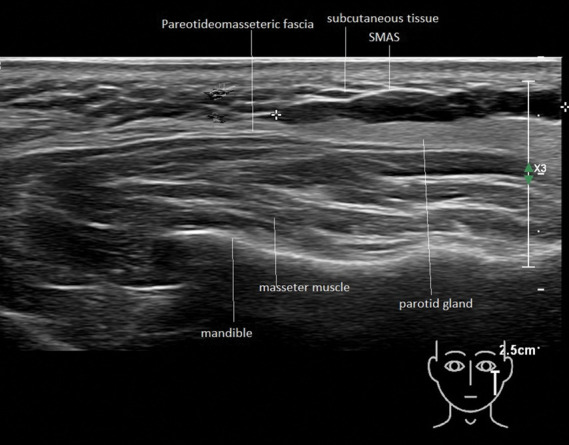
Case number 12. Hyaluronic acid filler deposit (between markers) injected in the lateral part of the left lower face. The ultrasound image displays a longitudinal anechoic tract. Presumably the material was meant to be injected in the subcutaneous plane. Clinically, a longitudinal nodular structure was visible.
